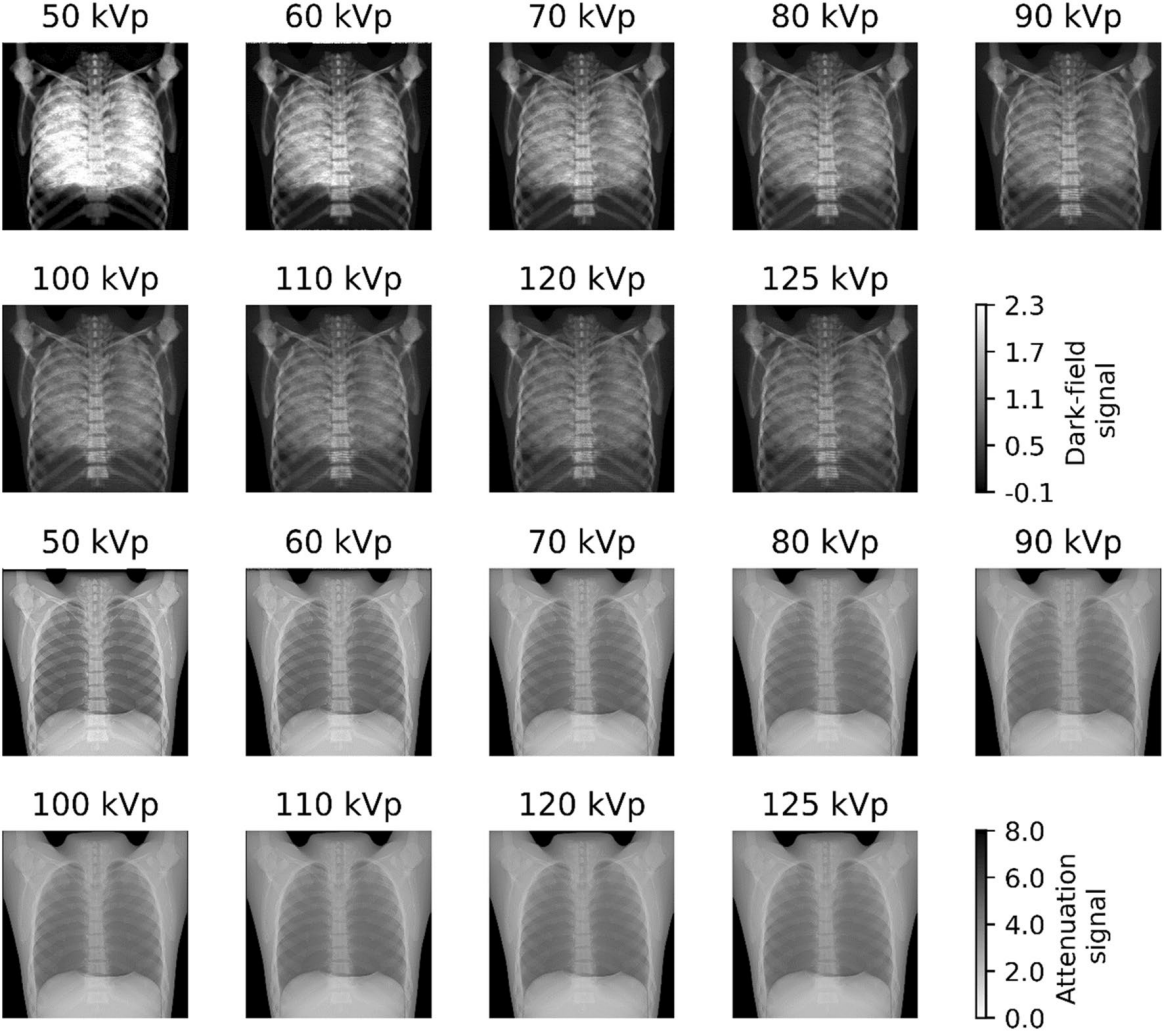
Dark-field (top) and attenuation radiograph (bottom) images acquired with different tube voltages. A distinct decrease in dark-field signal strength can be seen towards higher tube voltages. At lower tube voltages, a higher opacity of bone is present in attenuation radiographs, impairing the visualization of the “lung”.
